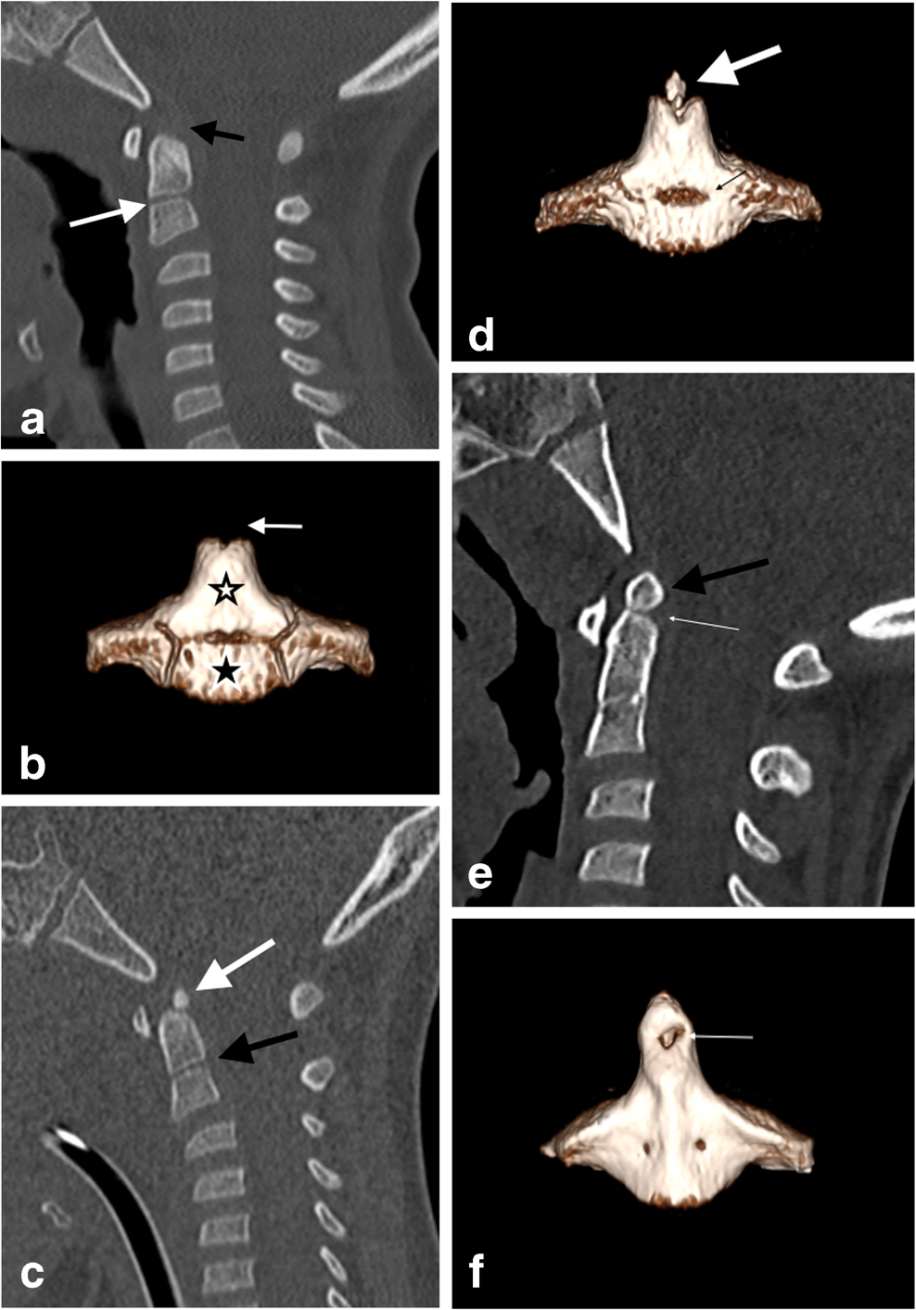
Progressive ossification of C2 vertebra: a: sagittal reconstructed CT image of the craniocervical junction of a 6-year-old male who fell 30 feet off a balcony—ossification has not yet begun in the chondrified apical dens (*black arrow*) in this 6-year old and the lower dental synchondrosis (*white arrow*) is also evident as non-ossified cartilage. C2-C3 pseudosubluxation is present.; b: volume-rendered three-dimensional reconstructed CT image of the C2 vertebra of the same 6-year-old male—the apical dens is non-ossified (*arrow*) and the ossified basal dental segment (*outlined asterisk*) and the body of the C2 vertebra (*black asterisk*) have not yet fused; c: sagittal reconstructed CT image of the craniocervical junction of a 5-year-old male who fell from a first floor window—ossification has begun but is incomplete in the chondrified apical dental segment (*white arrow*) and a thin ossifying lower dental synchondrosis (*black arrow*) is evident. The appearances should not be misinterpreted as fracture changes despite the high-energy clinical information apparent. C2-C3 pseudosubluxation is again present; d: volume-rendered three-dimensional reconstructed CT image of the C2 vertebra of the same 5-year-old male—the ossification of the apical dens is evolving (*white arrow*) and the lower dental synchondrosis is still visible (*thin black arrow*); f: sagittal reconstructed CT image of the craniocervical junction of a 6-year-old female who was a pedestrian struck by a car travelling at moderate speed—advanced ossification of the apical dental segment is present (*black arrow*) with early ossification across the upper synchondrosis (*white arrow*). Pseudosubluxation at the C2-C3 level is present; f: volume-rendered three-dimensional reconstructed CT image of the C2 vertebra of the same 6-year-old female—ossification across the upper synchondrosis (*thin white arrow*) and lower synchondrosis exhibits progressive features as union of the ossification centres proceeds
